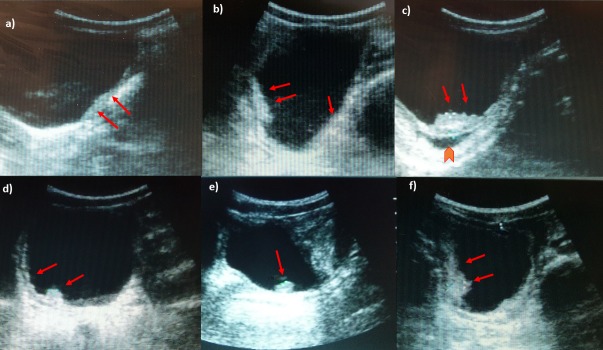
Ultrasonography images of urinary bladder of SAC infected with *S. haematobium*. a) Thickening of the bladder wall, transverse plane shows thickening of the left lateral wall (arrows); b) Diffuse thickening of the bladder wall more evident in the right posterior wall, echogenic snow in the lumen; c) In oblique longitudinal plane ultrasound image shows a mass-like lesion in the mucosa layer of the bladder. Block arrow indicates the dilation of the ureter; d) longitudinal plane shows a marked and diffuse thickening of the bladder wall with a mass-like lesion (arrow); e) mass-like lesion in the absence of a marked and diffuse thickening of the bladder wall; f) multifocal thickening of the wall, particularly evident on the right and posterior wall.
